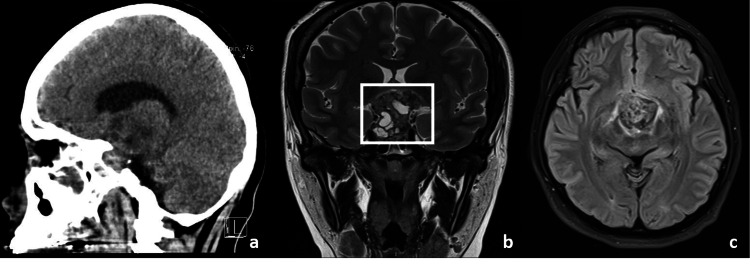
Imaging findings of case three. Sagittal computed tomography, coronal magnetic resonance imaging in T2, and a transversal fluid-attenuated inversion recovery (a, b, and c respectively) images show a sellar mass with multiple hypointense and hypodense areas, as well as with different densities in its content with walls that limit hyperintense content within a solid content (square).
